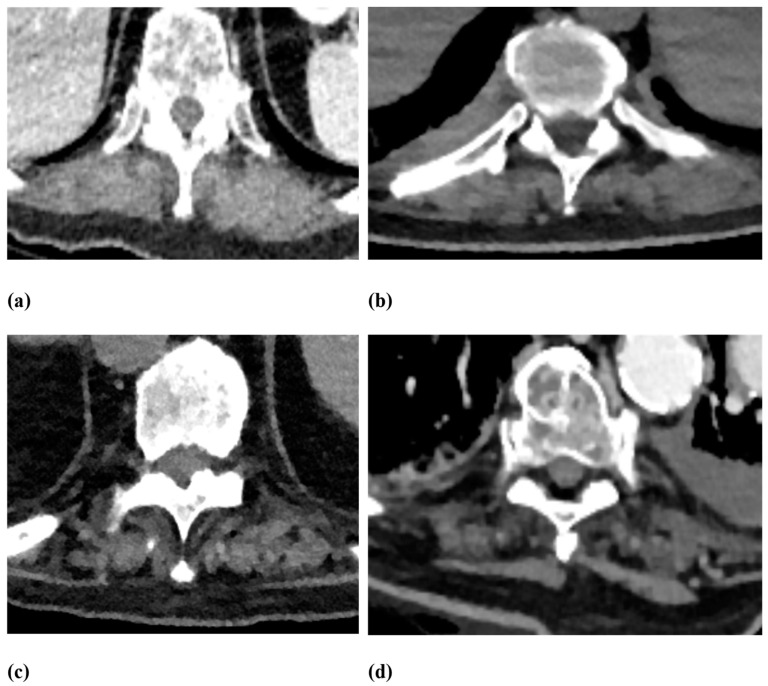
Goutallier score modified for CT scans along the thoracic spine. (a) Grade 0 for normal muscle without fat. (b) Grade 1 for a few fatty streaks within the muscle. (c) Grade 2 for less fat than muscle within the muscle. (d) Grade 3 for approximately the same amount of fat and muscle within the muscle.
